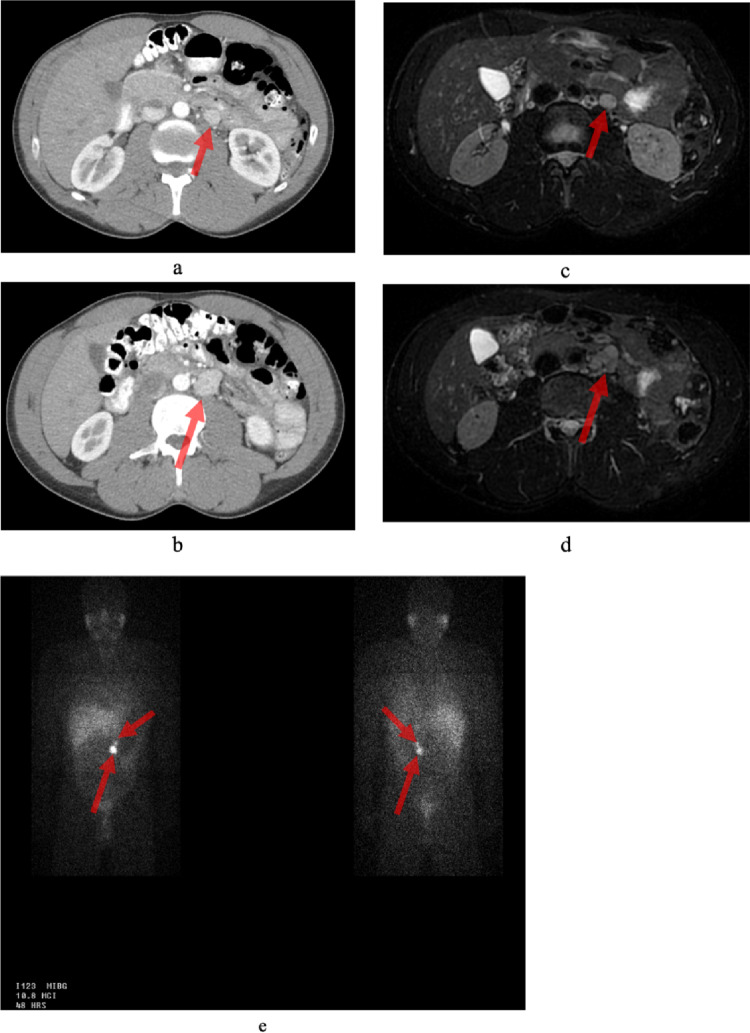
Two left retroperitoneal paragangliomas are demonstrated on CT, MRI, and I-123 metaiodobenzylguanidine (MIBG) scans. On CT (a, b), 2 avidly enhancing soft tissue masses (small and large red arrows) are identified in the left retroperitoneum with the larger lesion at the level of the IMA likely originating from the organ of Zuckerkandl. Both lesions are hyperintense on fat-suppressed T2-weighted axial images (c, d, small and large red arrows) and demonstrate increased uptake on a I-123 MIBG scan (e, small and large red arrows). Both were pathologically proven paragangliomas
